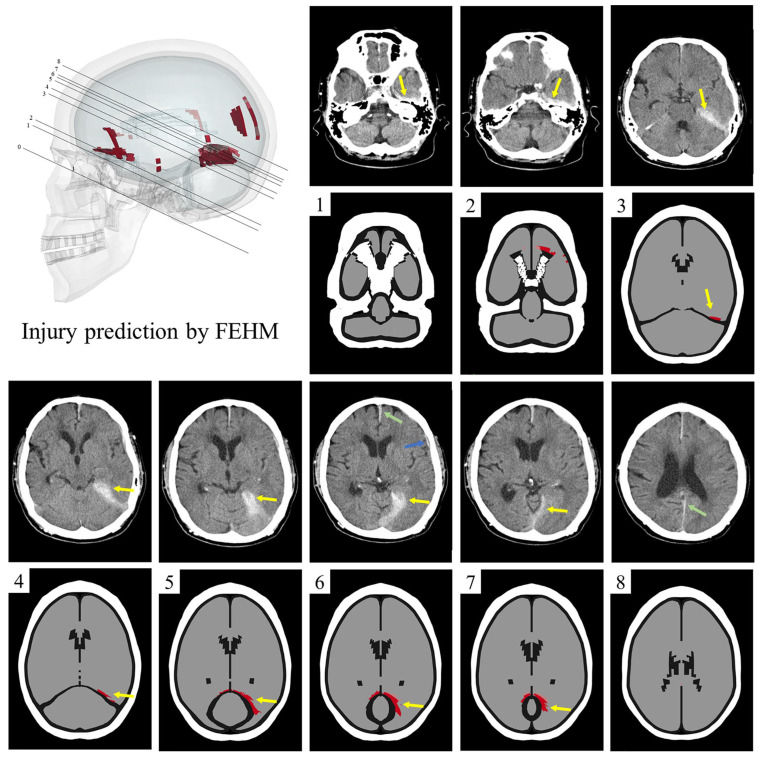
The comparison of injury areas in FEHM and CT images. Clinically, there is hematoma or contusion from the temporal lobe to the upper tentorium (yellow arrow), as well as hemorrhage in the falx cerebri, between the cerebral hemispheres (green arrow), and contusion in the sylvian fissure (blue arrow). The FEHM injury predictions of the corresponding sections are below the CT images. In sections 3–7, the yellow arrow indicates the injury above the tentorium, which corresponds to the clinical results. In addition, injuries in the orbitofrontal region are also indicated (section 2), which are not present in CT images. Hemorrhaging between the cerebral hemispheres and contusions in the sylvian fissure are visible only in the CT images (sections 6 and 8).
